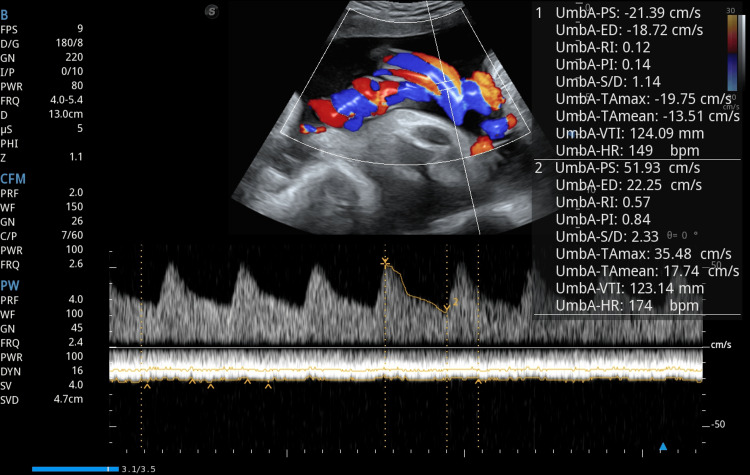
Umbilical artery Doppler ultrasound demonstrating waveforms and blood flow parameters in a fetus at 32 weeks of gestation
